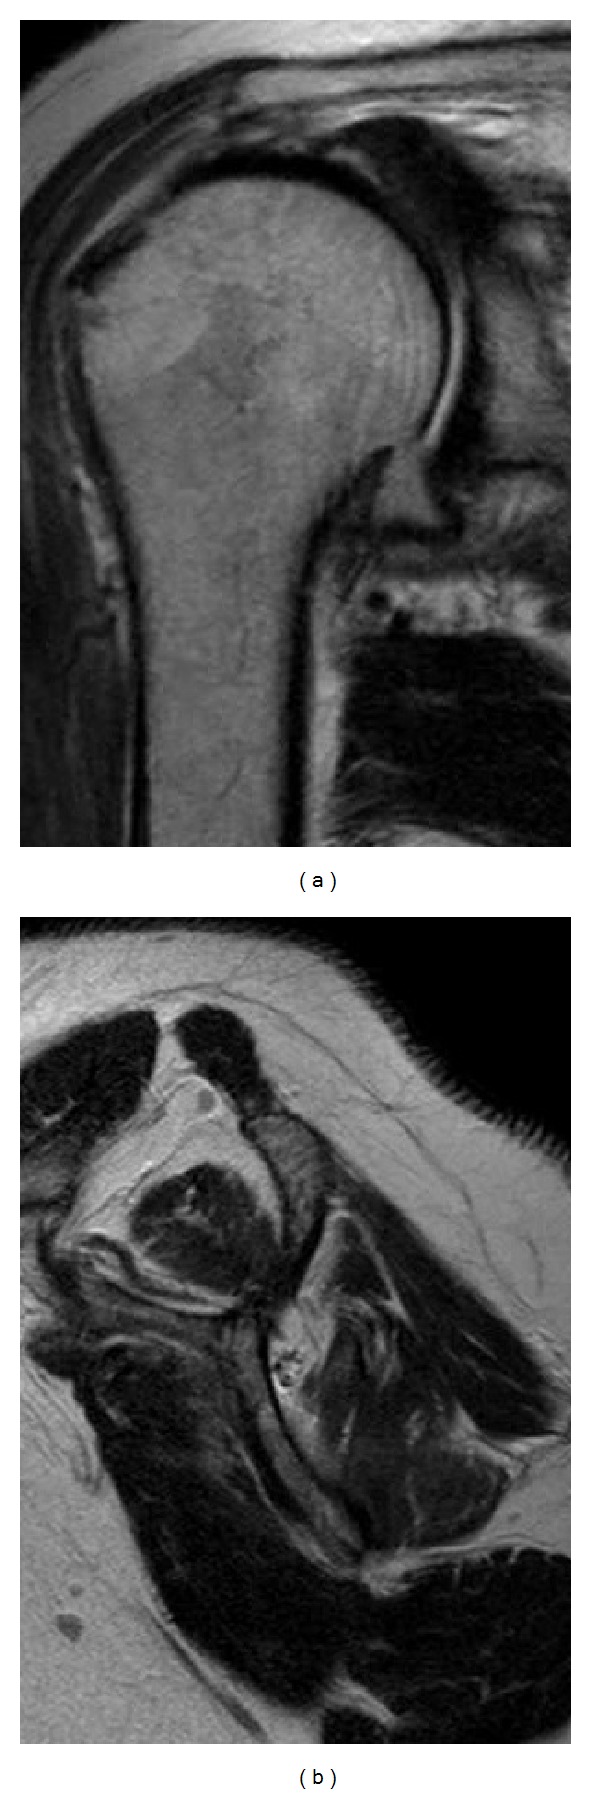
MRI imaging upon initial presentation to our hospital. (a) Coronal view of T2 shows a large tear approximately 3 cm long in the supraspinatus tendon. (b) Oblique sagittal view of T2 shows atrophy and fatty degeneration of the supraspinatus muscle.
